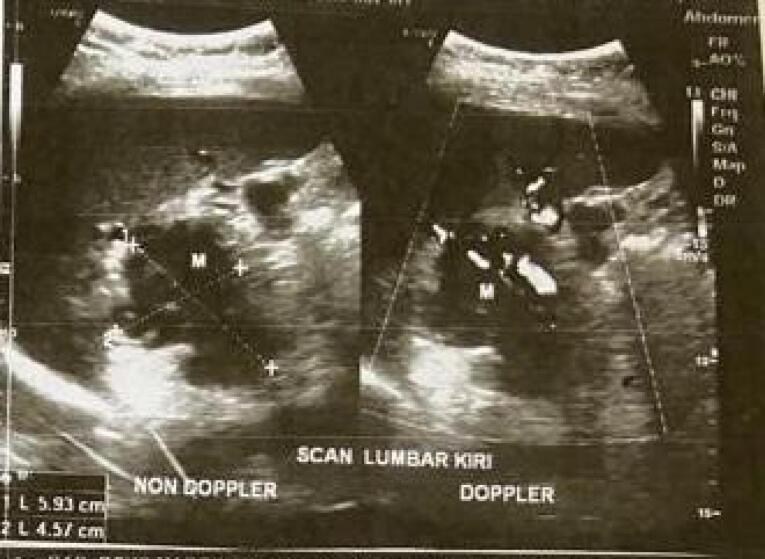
The patient underwent a CT scan, which revealed the presence of a cauda pancreatic solid-cystic mass measuring 12 cm × 10 cm. During the surgical procedure, the surgeon selectively excised a portion of the pancreatic tissue and thereafter submitted it for anatomical pathology examination. The anatomical pathology examination identified the presence of a lump in the stomach, which was the first tissue preparation for a pancreatic head tumor. The subsequent tissue of the curvatora minor lymph gland was also conducted. Macroscopic clinical diagnosis revealed a pancreatic tumor with two initial tissue fragments of 1 cm × 1 cm × 0.5 cm, with a brownish-white coloration and a spongy texture. The second tissue comprised a tissue with dimensions of 2.5 cm × 1.5 cm × 1 cm, enclosed by a white, sticky capsule.
